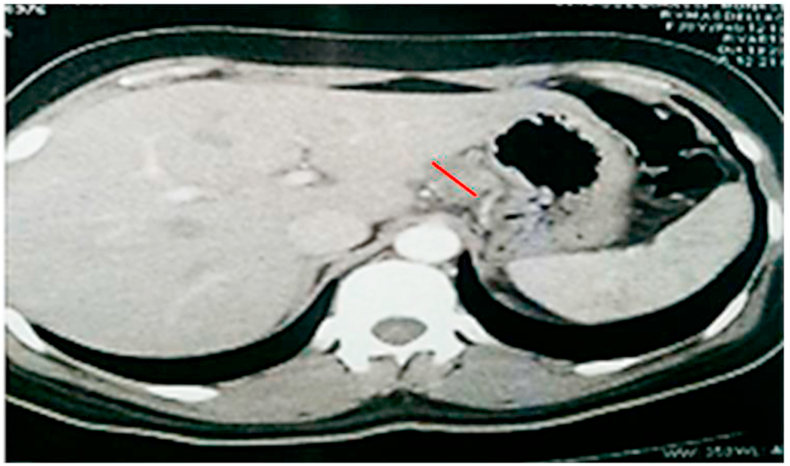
Abdominal Computed tomography (CT) revealed a 45 × 28 mm low-density lesion, arising from the pancreatic body.
